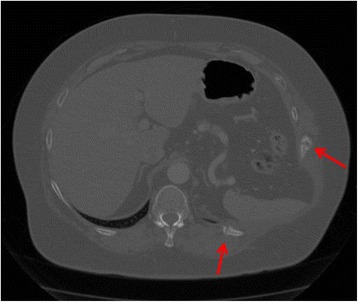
Axial computed tomography image displayed in a bone window. The image shows left lateral 10^th^ and left posterior 11^th^ rib fractures (*arrows*)
